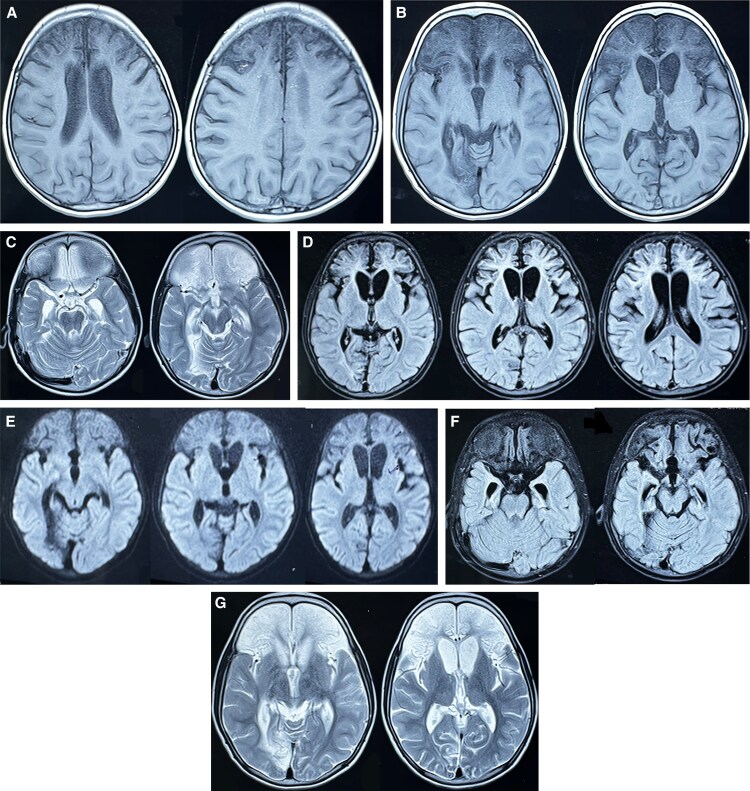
(*A,B*) T1 images show hypointensities with cortical atrophy in the bilateral frontal and occipital lobes with ex vacuo dilation of ventricles, including the prominence of ventricles. (C–G) T2/FLAIR images indicate encephalomalacic changes with surrounding gliosis in the bilateral frontal and right occipital lobes as sequelae of an old insult.
